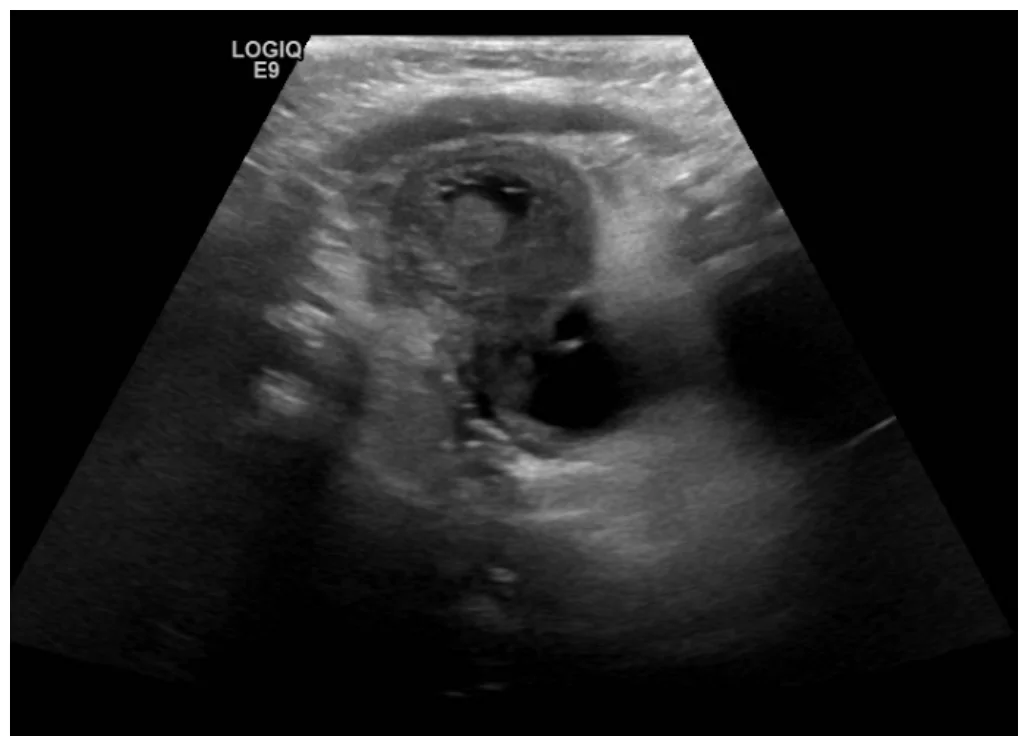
Pre-nephrostomy bladder ultrasound obtained during the acute postoperative deterioration.
